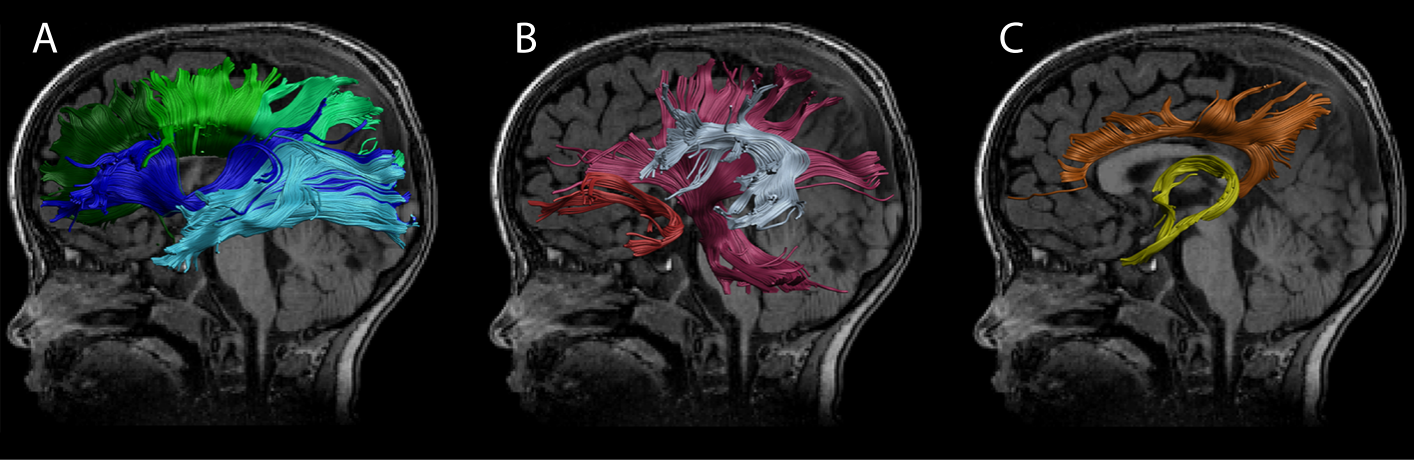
Isolated white matter tracts. Diffusion measures were calculated for 10 unique white matter tracts; a) dark green: genu of corpus callosum, lime green: body of corpus callosum, mint green: splenium of corpus callosum, dark blue: left inferior fronto-occipital fasciculus (IFO), light blue: left inferior longitudinal fasciculus (ILF); b) pink: left pyramidal, silver: left superior longitudinal fasciculus including the arcuate fasciculus (SLF), red: left uncinate fasciculus (UF); c) orange: left cingulum bundle, yellow: fornix. Tracts are shown on a T1-weighted image from a male 3.7 years of age
